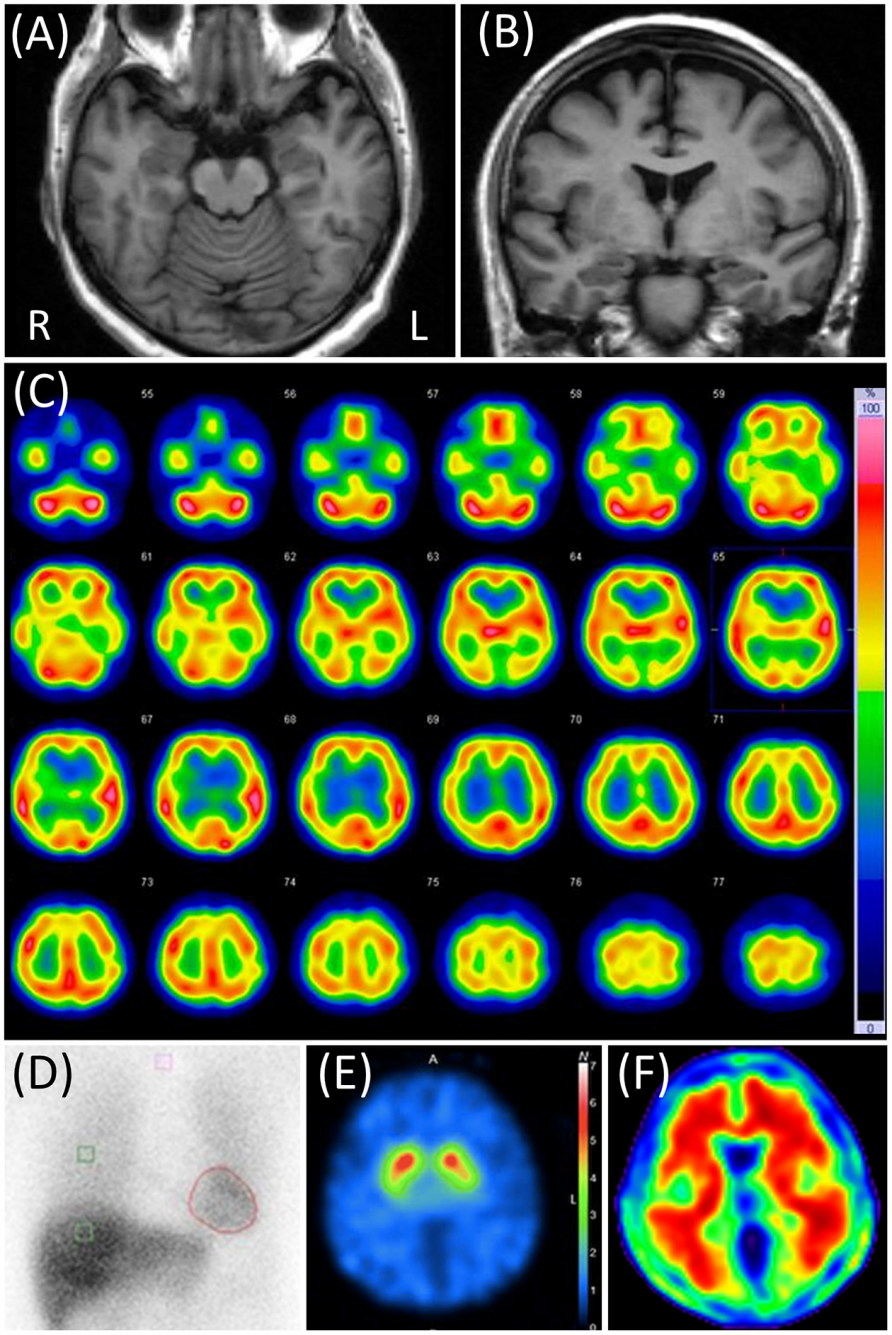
Case 1 images. (A,B) Brain magnetic resonance T1-weighted images. None of them showed any abnormalities. (C) N-isopropyl-p-[123I] iodoamphetamine (123I-IMP) single-photon emission computed tomography (SPECT) not showing any particular abnormalities. (D) Cardiac [123I]-metaiodobenzylguanidine scintigraphy. Heart-to-mediastinum ratios were 3.33 and 2.28 in early and delayed phases, respectively. The washout ratio was 48.8%. (E) 2β-carbometoxy-3β-(4-iodophenyl)-N-(3-fluoropropyl) nortropane (FP-CIT) SPECT. The specific binding ratios were 6.53 and 6.04 in the right and left striatum, respectively. The asymmetry index was 7.9%. (F) Florbetapir 18 positron emission tomography showing positive results.
